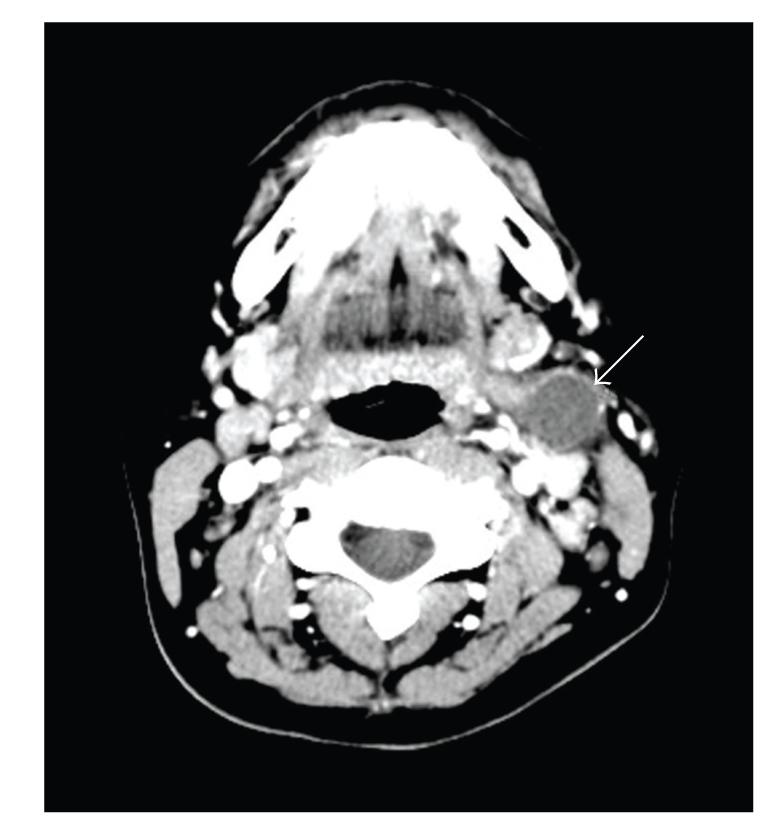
Axial contrast-enhanced CT. A 2 cm well-circumscribed, low attenuation mass (arrow) is present at the expected location (jugulodigastric (Level 2) lymph node or second branchial cleft cyst).
